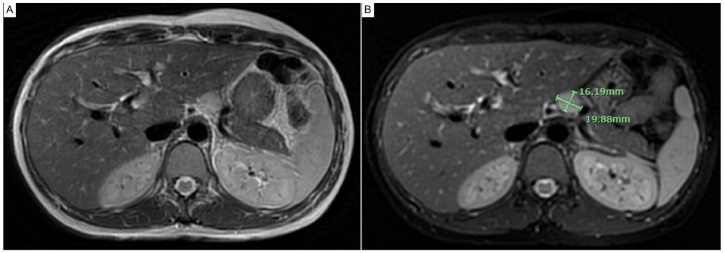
(A) Magnetic resonance imaging scan of pancreatic insulinoma: axial T2-weighted image alongside T2-weighted image employing fat suppression, (B) revealing a distinct, homogeneous mass at the superior aspect of the pancreas (approximately 2.0 × 1.6 cm).
